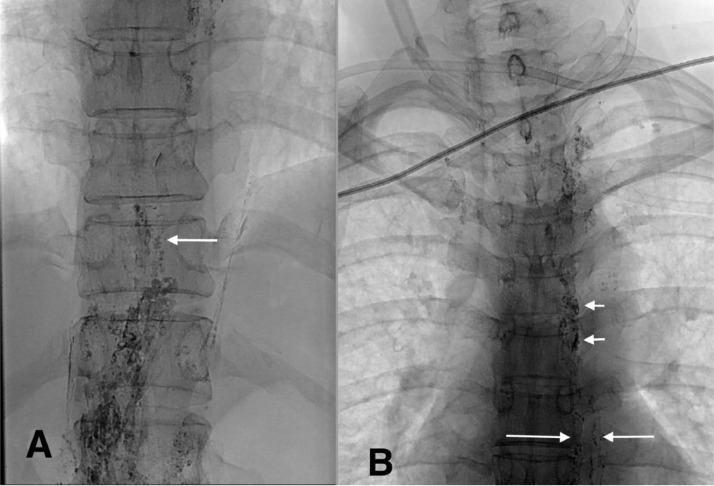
Radiography 8 days after thoracic duct (TD) embolization. A) Glue was completely washed out from the distal part of the TD (arrow). B) Some glue could be observed in the middle segment of the TD and its branch (long arrows), and minimal stagnation of the contrast agent was observed in the TD (short arrows).
